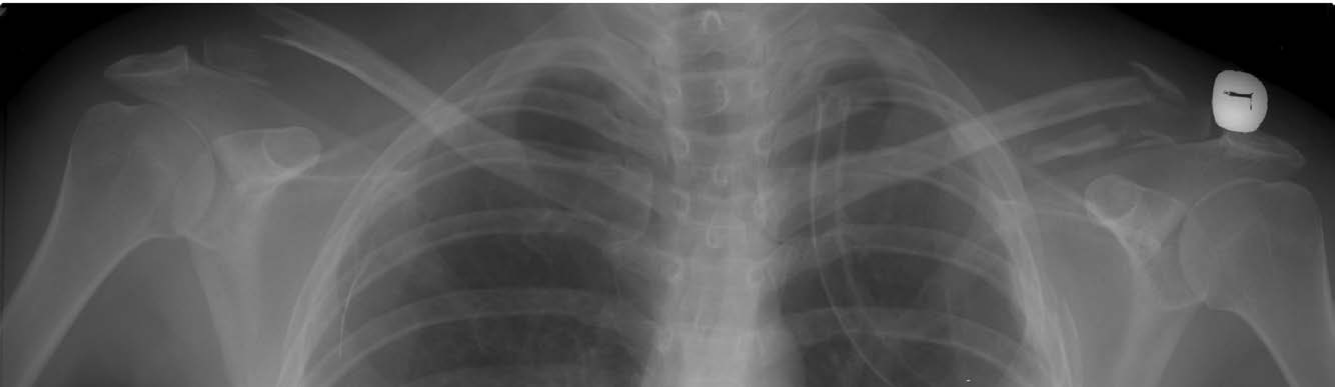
Preoperative bilateral shoulder radiography image.
